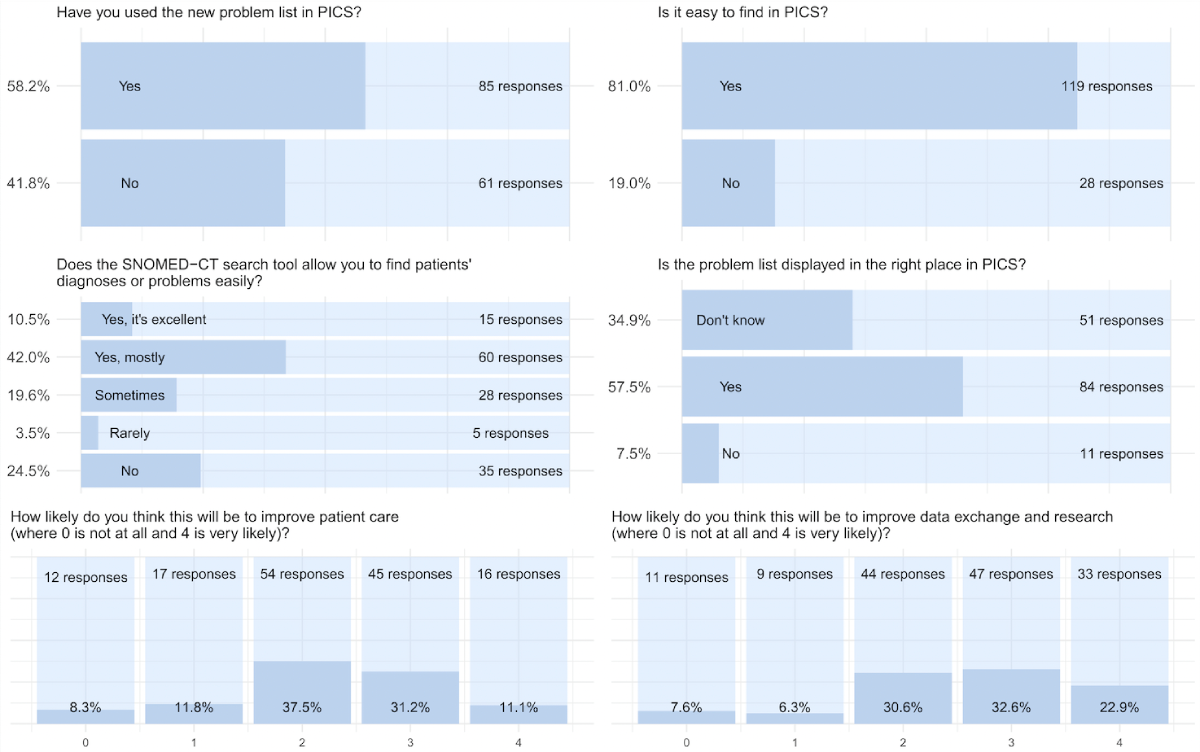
Questionnaire summary for user feedback. PICS: Prescribing Information and Communication System; SNOMED-CT: Systematized Nomenclature of Medicine–Clinical Terms.
